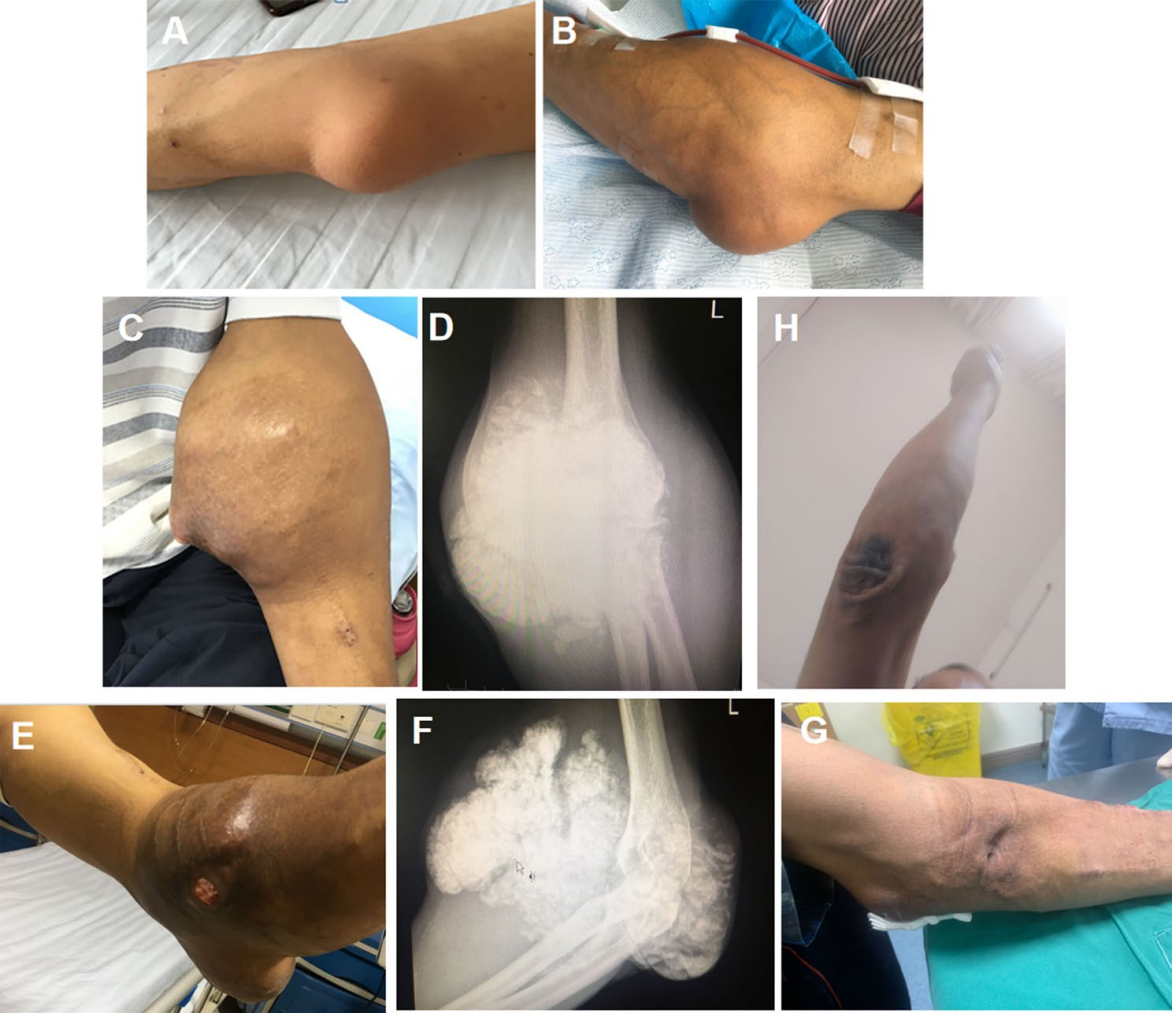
Evolution and Management of a Left Elbow Mass in a Uremic Patient. (A) Photograph from 2016 showing an initial nodular mass on the left elbow joint, with intact skin and no signs of inflammation. (B) By 2017, the mass had significantly enlarged. C, D) Gross appearance and X-ray images of the left elbow mass in 2018. E, F) Gross appearance and X-ray images of the left elbow mass in 2021. G) By March 2024 (two months after intensified phosphorus-lowering treatment), significant resorption of the mass was observed, accompanied by complete healing of the ulcer and sinus tract. H) As of June 2, 2025, the UTC lesion in the patient’s left elbow had been completely resorbed
